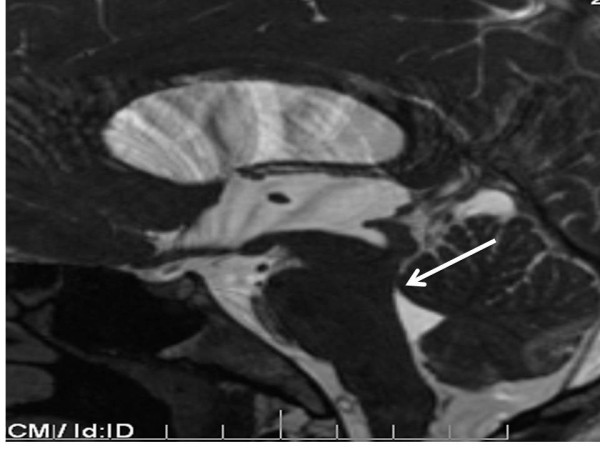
Midsagittal T2-weighted MRI of his head showing hydrocephalus with lack of cerebral aqueduct patency (*arrow*).
